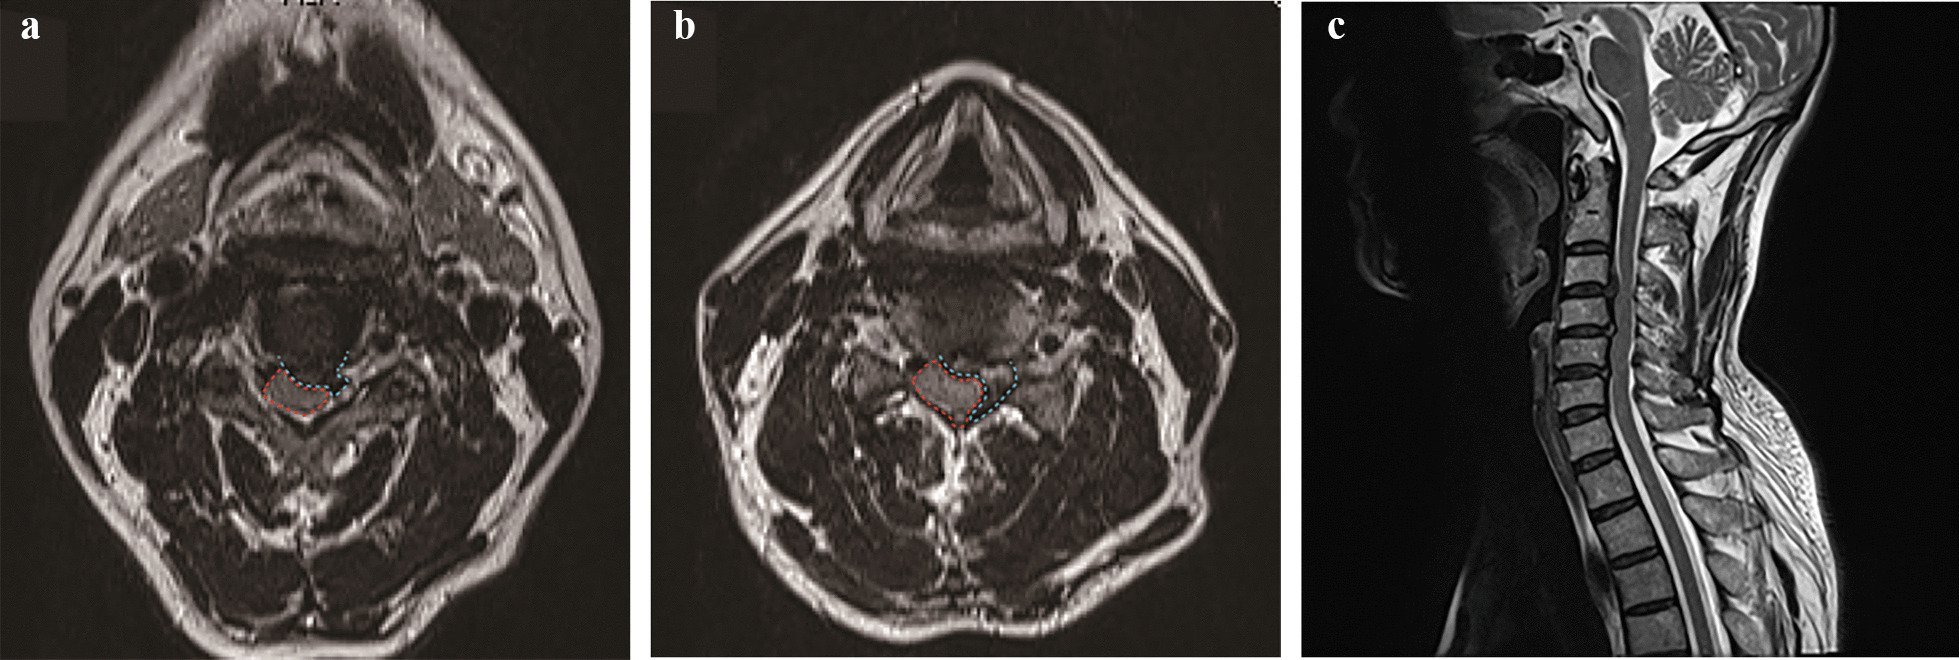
Preoperative MRI images of the cervical region of the patient. a T1-weighted axial view showing the herniation of the disc (blue line) to the anterior aspect of the spinal cord (red line) at the C3/4 level; b T1-weighted axial view showing the herniation of the disc (blue line) to the lateral-posterior aspect of the spinal cord (red line) at the C4/5 level; and c T2-weighted sagittal view showing the spinal cord of the patient being compressed by the herniated disc
